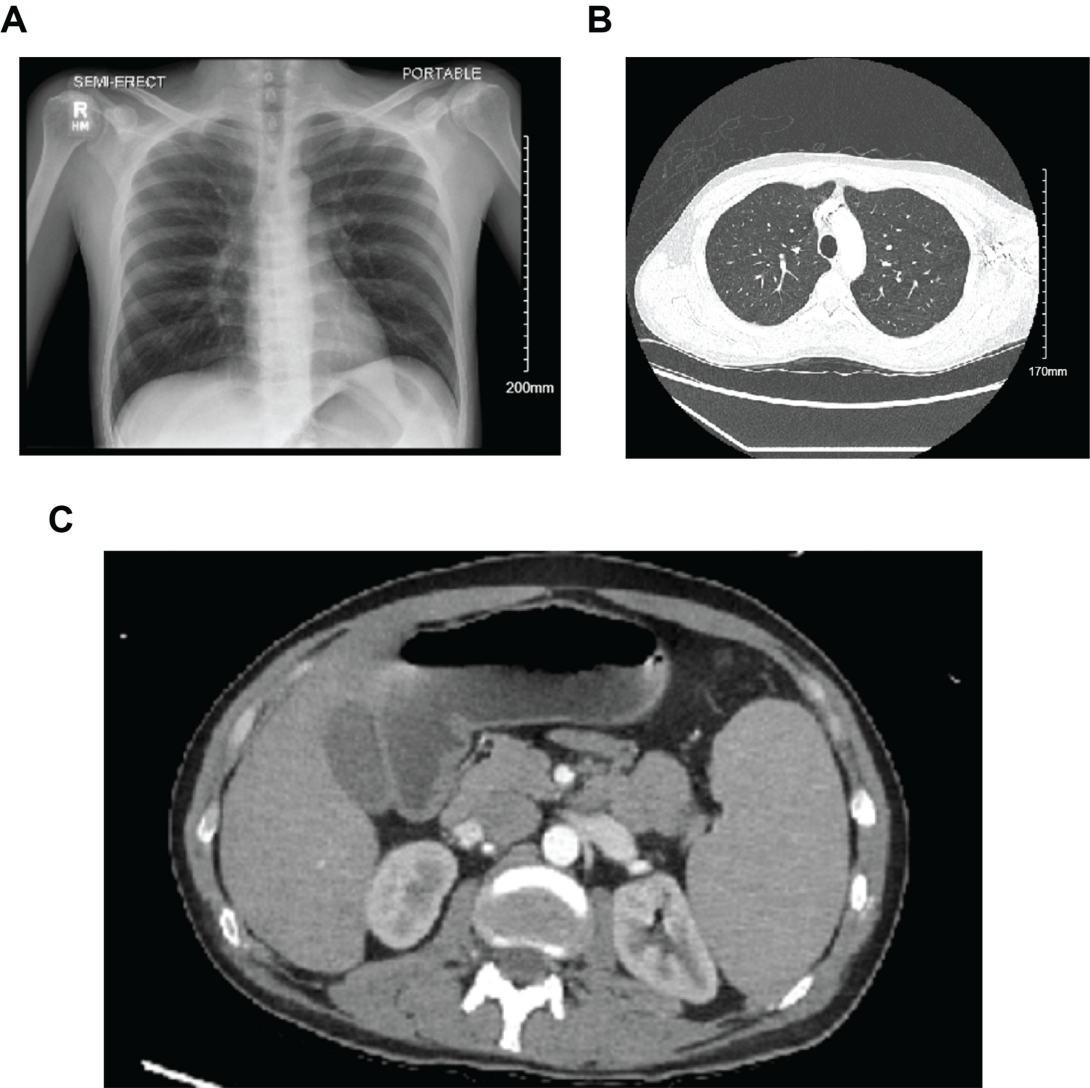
Unremarkable initial chest X-ray-PA view (A), computed tomography (CT) angiogram of the chest-axial view (B), and CT abdomen and pelvis-axial view (C) PA: posteroanterior
